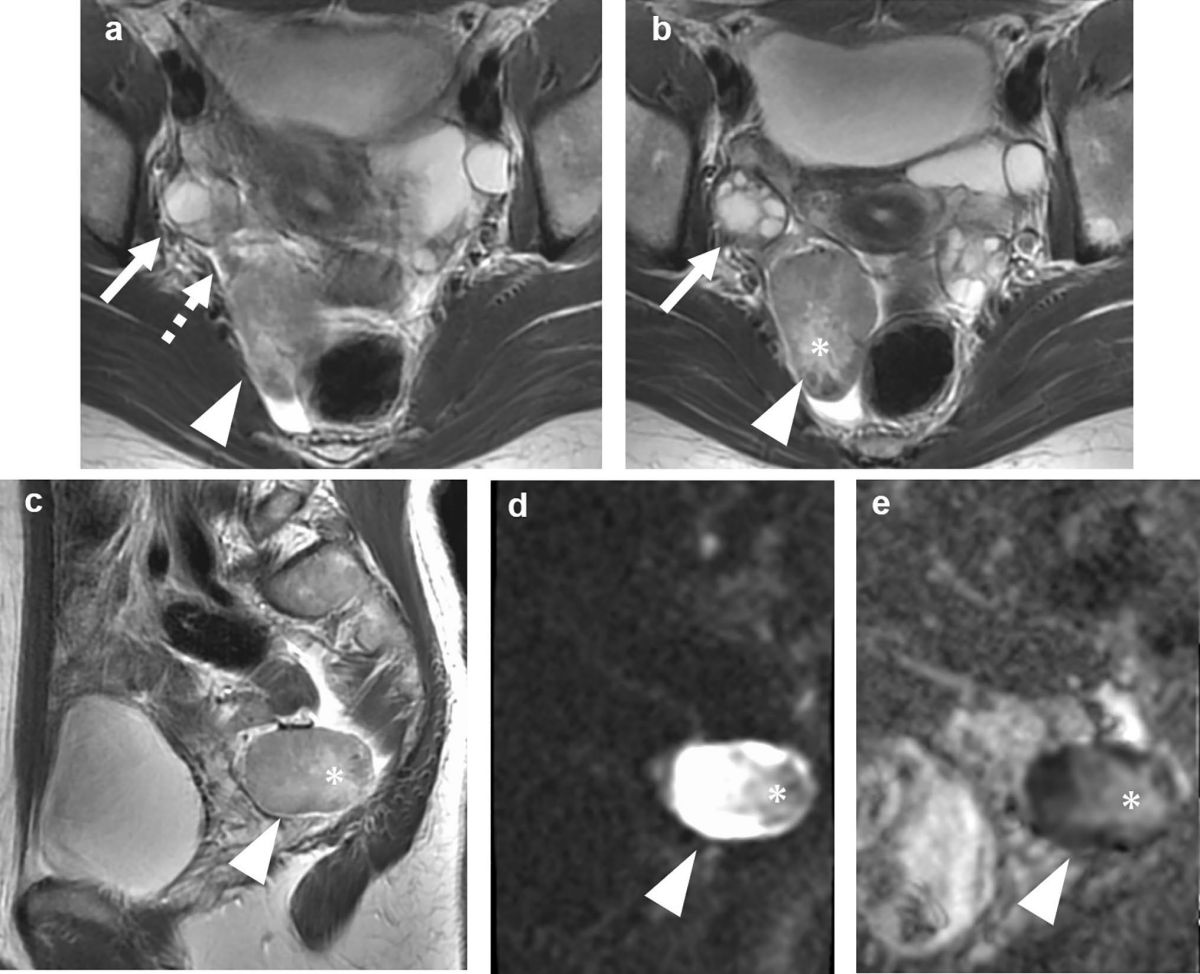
A 27-year-old woman presented with right lower abdominal pain of acute onset. a Axial T2-weighted MR image shows a well-defined solid tumor (arrowhead) posterior to both the uterus and right ovary (arrow), associated with twisted structure between the tumor and right ovary (dashed arrow). b Another axial T2-weighted image of caudal level shows a central area of increased intensities (asterisk), which is suspected of necrosis caused by torsion. The rest of the tumor is intermediate intensities. c Sagittal T2-weighted image shows tumor of intermediate intensities (arrowhead) containing areas of increased intensities suggestive of necrosis (asterisk). d Diffusion-weighted image at b-value of 800 shows the tumor of high intensities (arrowhead) except for areas of decreased signals in necrosis (asterisk). e ADC map image shows decreased ADC value (1.0 × 10^–3^ mm^2^/s) in the solid component except for areas suspected of necrosis of increased ADC value (2.3 × 10^–3^ mm^2^/s) (asterisk)
